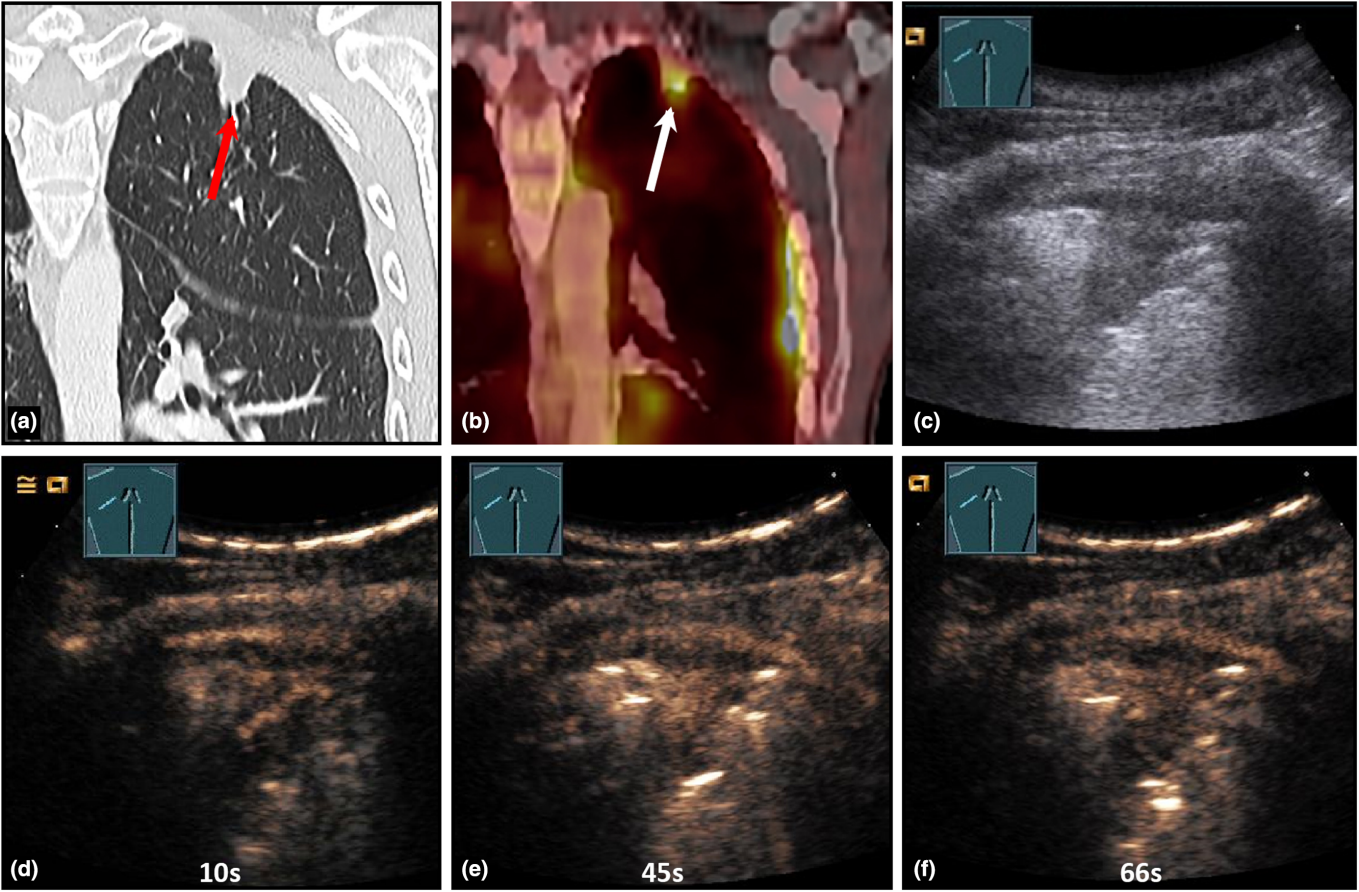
A 39‐year‐old female patient was admitted with unexplained left‐sided pleural effusions and respiratory pain. The computed tomography (courtesy of Prof. Dr. Andreas H. Mahnken, Department of Radiology, University Hospital Marburg) shows a lung consolidation in the left upper lobe (a). The lesions reveal an increased Fluorodeoxyglucose uptake on positron emission tomography computed tomography (courtesy of Prof. Dr. Markus Luster, Department of Nuclear Medicine, University Hospital Marburg) (b). B‐mode ultrasound shows a hypoechoic lung consolidation in the left upper lobe (c). On contrast‐enhanced ultrasound, after 10 s, the lesion shows an inhomogeneous, bronchial‐arterial pattern of enhancement with small non‐perfused areas (d); after 45 s, the lesion still shows an inhomogeneous enhancement (e); after 66 s, the lesion shows a decrease of enhancement (rapid washout) (f). Ultrasound‐guided biopsy showed a granulomatous inflammation. With a positive QuantiFERON‐TB Gold test, a diagnosis of tuberculous pleuropneumonia was made.
